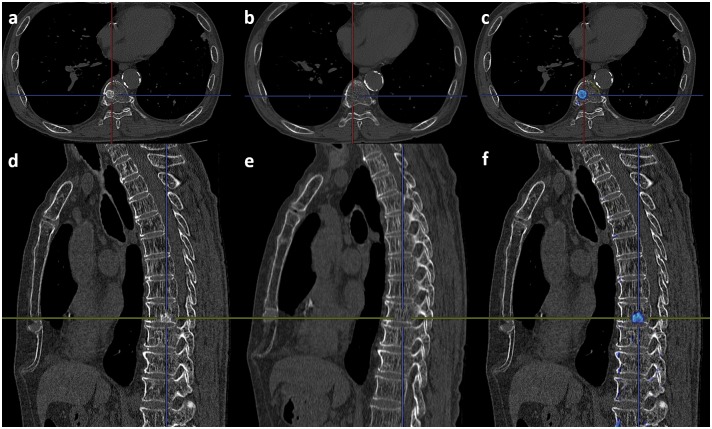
A case of osteoblastic metastasis in the body of the ninth thoracic vertebra. (a) Follow-up axial image. (b) Initial axial image. (c) Result of the 3D-CT subtraction. The blue color indicates the metastasis. (d) Follow-up sagittal image. (e) Initial sagittal image. (f) Result of the 3D-CT subtraction. The blue color indicates the metastasis.
